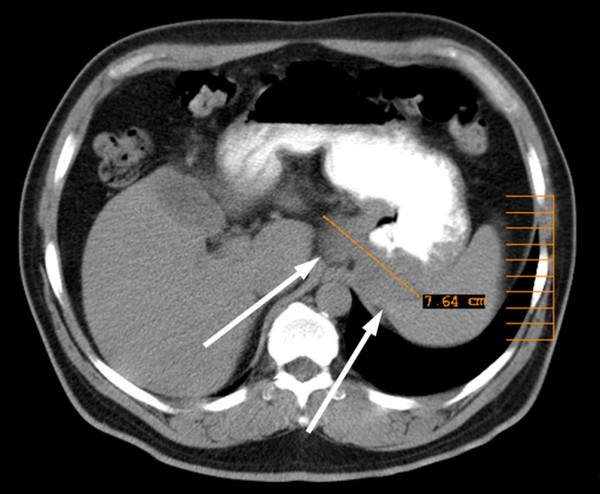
Initial CT-Scan. Initial CT scan showing the advanced tumor of the esophagogastric junction before starting neoadjuvant therapy.
